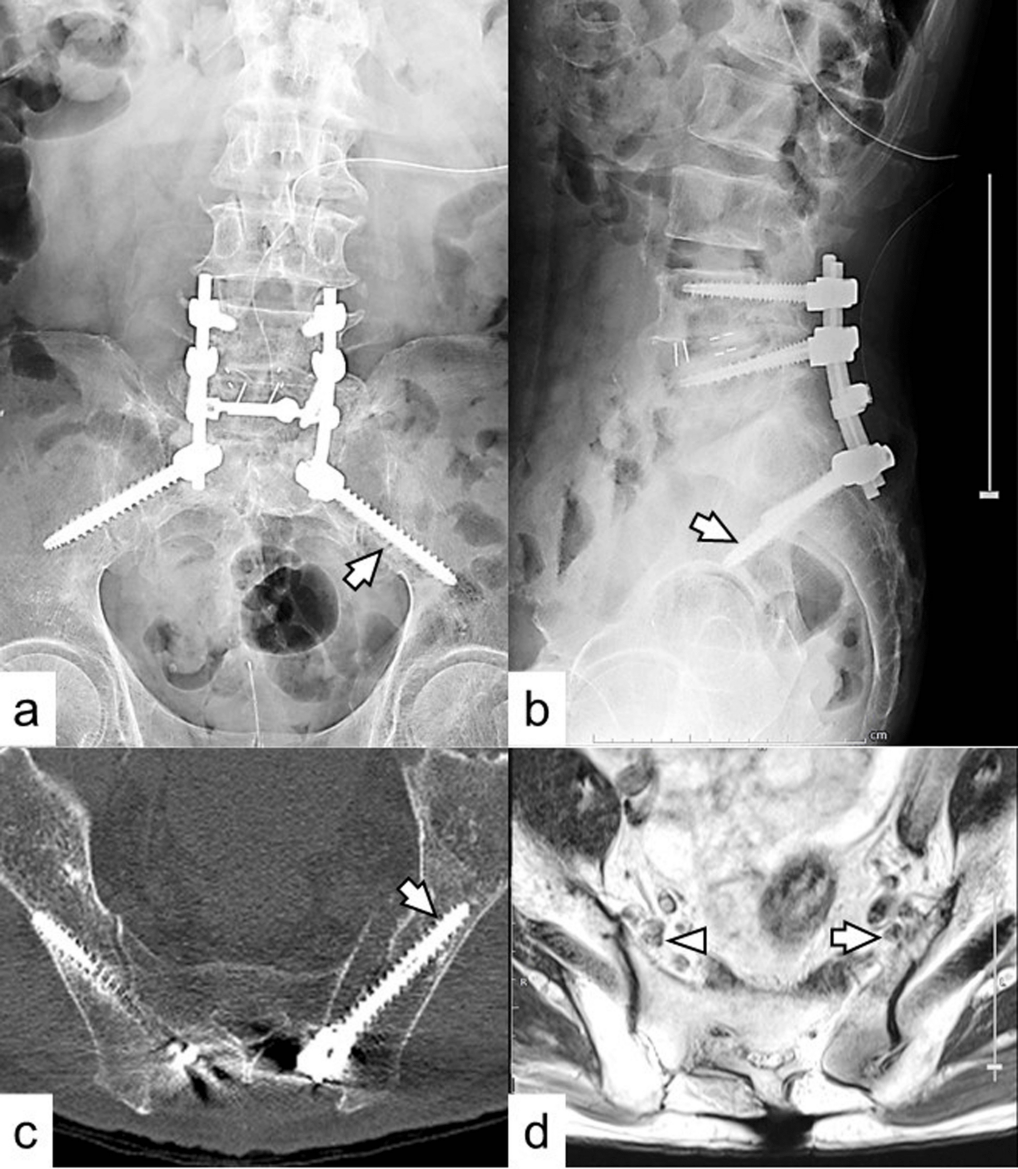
Postoperative images. Anteroposterior (a) and lateral (b) views of the plain radiography and CT (c) reveal the replaced left S2AI screw in the correct trajectory (arrows). The right S2AI screw was also replaced with a larger diameter screw because of slight loosening. (d) T2-weighted MRI revealed that the left L5 spinal nerve had returned to its original location (arrow) compared to the preoperative image (Figure 1d), and there was no difference in the position of the right L5 spinal nerve (arrowhead). S2AI, S2 alar-iliac; CT, computed tomography; MRI, magnetic resonance imaging
